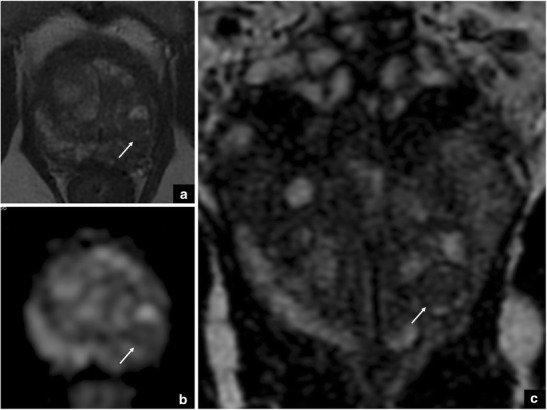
The arrows show a focal nodule bulging in the left peripheral zone, characterised by low signal intensity on axial (a) and coronal (c) T2-weighted imaging, with sharply defined margins, and restricted diffusion on the ADC map (b). The presence of tiny bright spots (corresponding to dilated acini) is consistent with a nodule of stromal benign prostatic hyperplasia (BPH), which may sometimes protrude from the central zone
